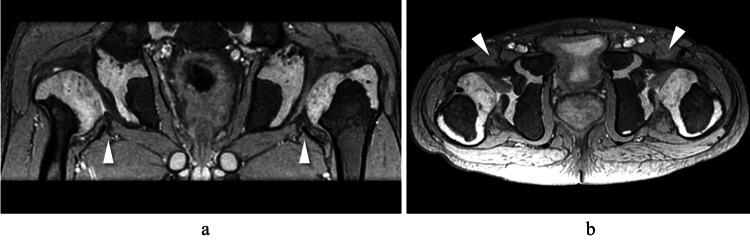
MRI T2*-weighted images at age 8. (a) Coronal view: Progressive lateral displacement of the femoral head is observed (white arrowheads). (b) Axial view: Hypertrophied ligamentum teres and limbus are identified (white arrowheads).
